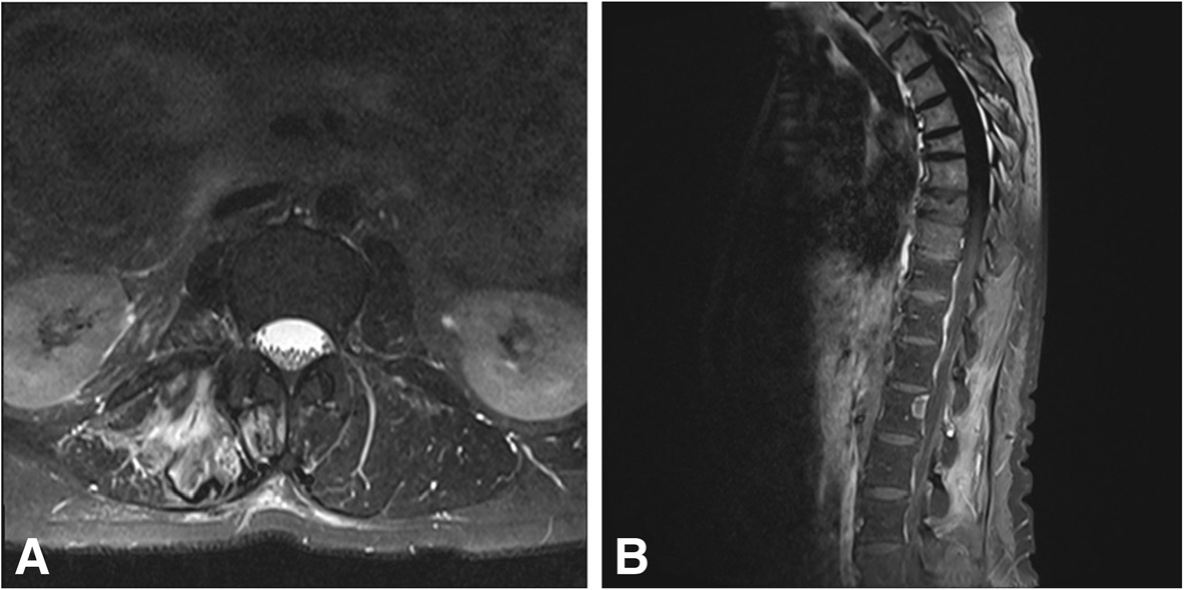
Six-month follow-up contrast-enhanced MRI confirmed complete tumour removal in axial (A) and sagittal (B) planes.
